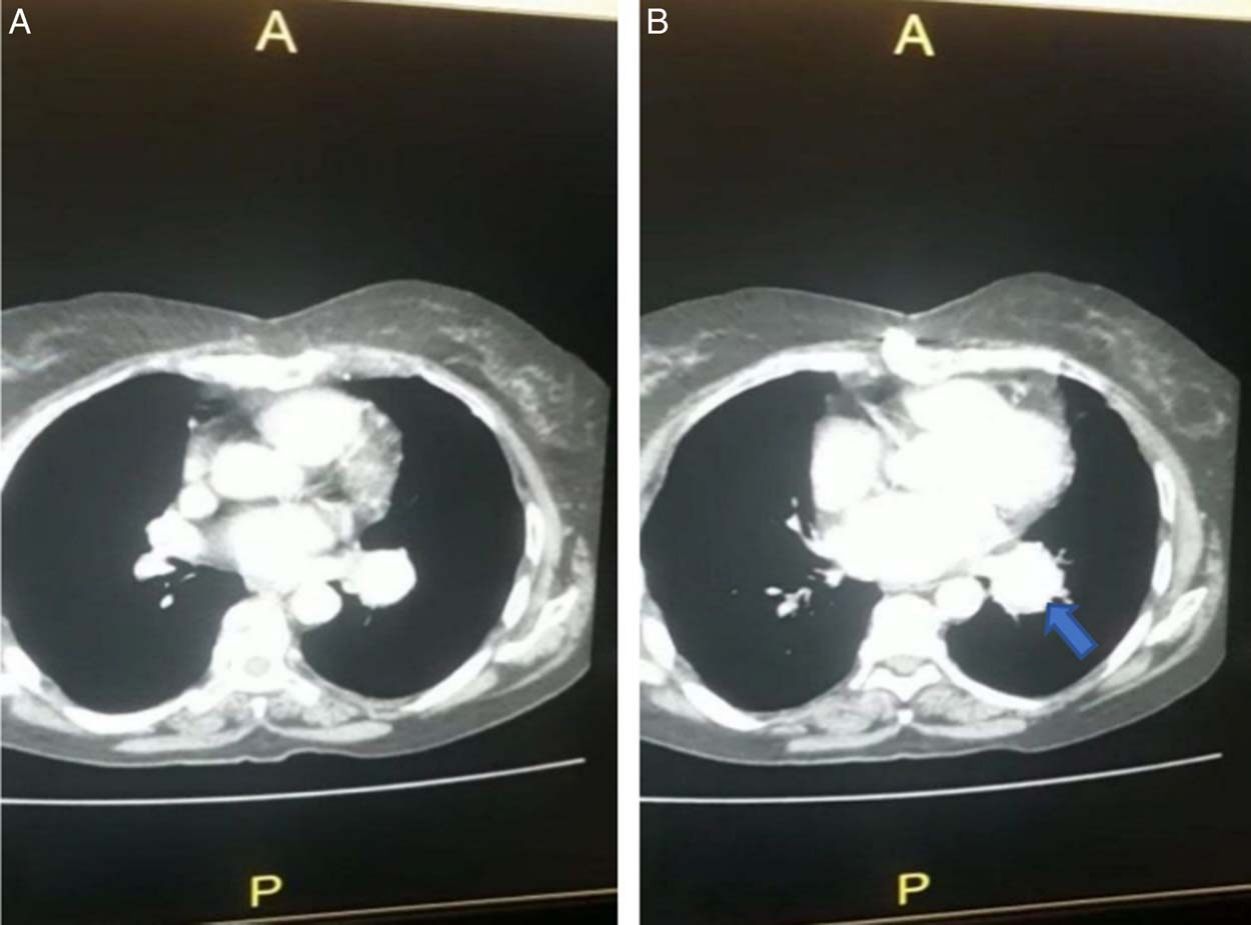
(A, B): Computed tomography pulmonary angiogram showing a lesion in the hilum of the left lung measuring 37×52 mm and multiple cavitary consolidations in direct contact with the segmental branch of the left pulmonary artery in the lower left lobe representing pulmonary artery aneurysm (arrow).
